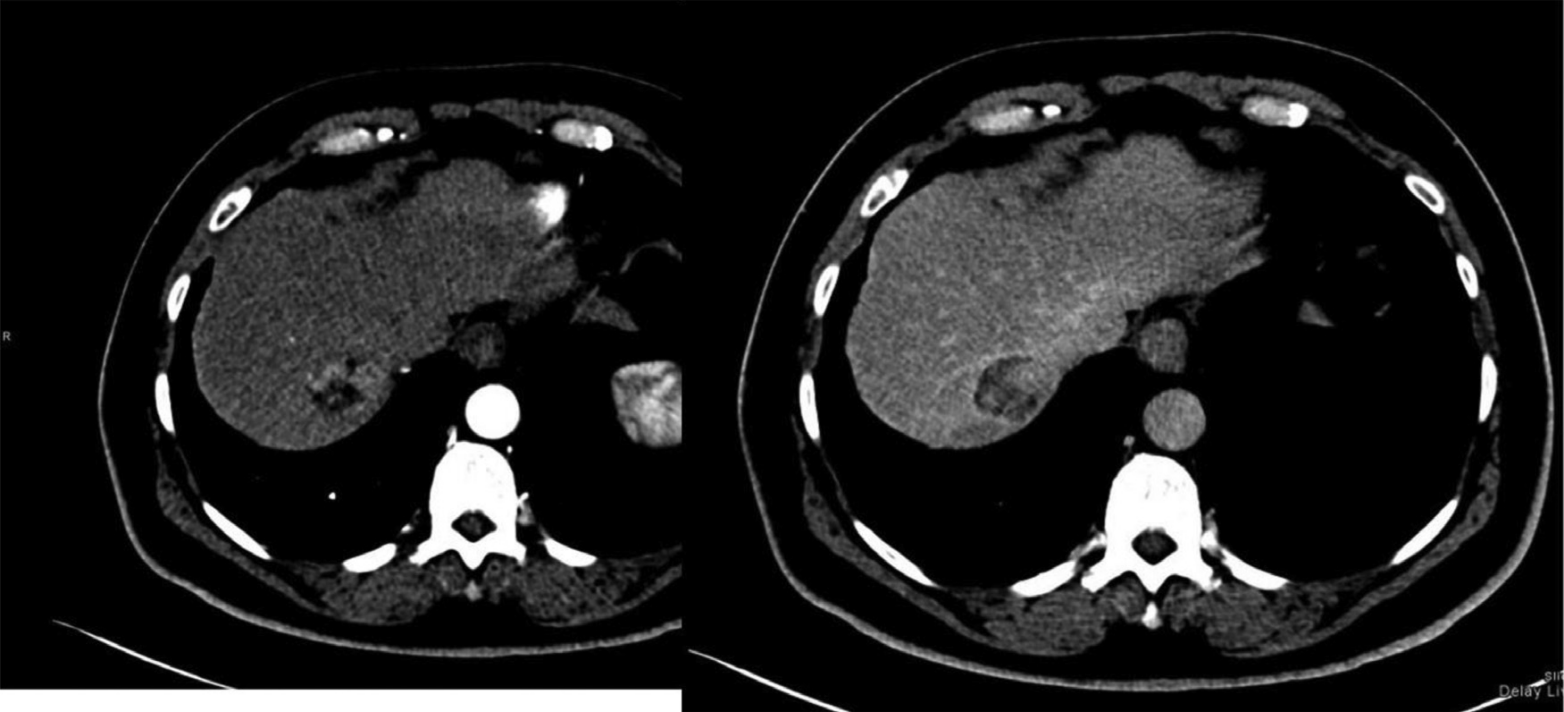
Segment VII liver HCC. Abdominal enhanced CT axial images in the arterial (A) and delayed phases (B). A 3.7-cm segment VII liver lesion with arterial enhancements and delayed washout with capsular enhancement compatible with HCC.
